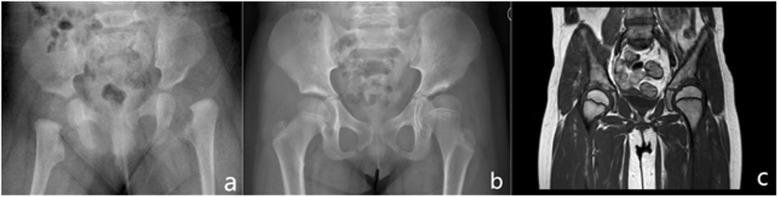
a Pre-operative anteroposterior radiograph of a 4-month-old girl with IHDI type III dislocation of the left hip. b 5.5 years after surgery, there were signs of acetabular dysplasia in the left hip on X-ray. c MRI showed sufficient cartilage acetabular coverage in the coronal plane
